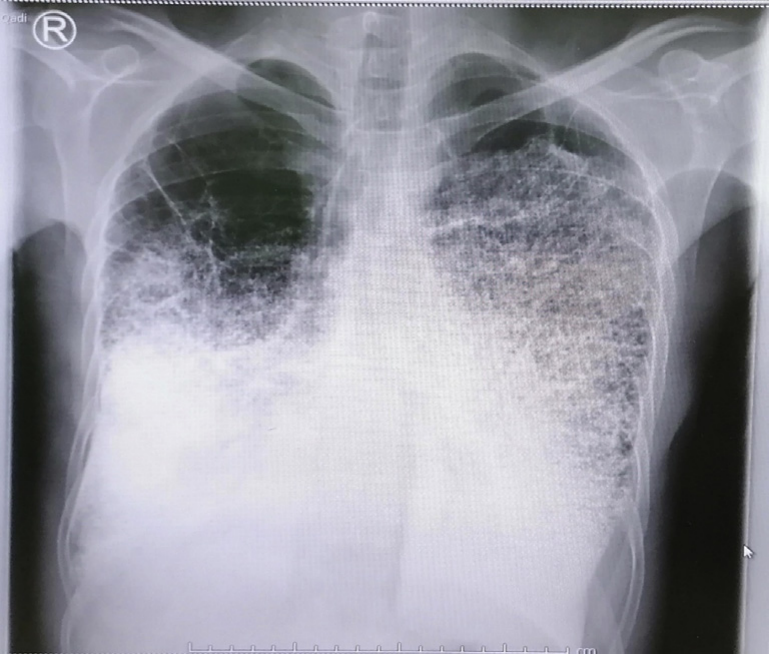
Chest x-ray with bilateral reticulonodular shadowing.
